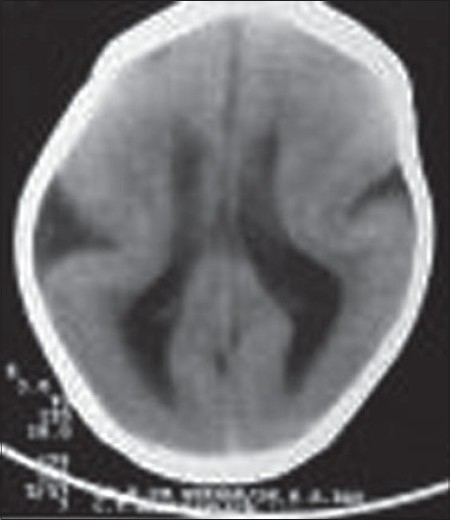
Noncontrast axial CT scan showing the presence of lissencephaly with pachygyria-agyria complex
